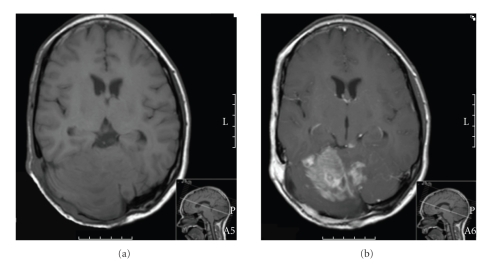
T1-weighted axial MRI-scan of the brain. T1-weighted axial MRI showed a (sub)occipital right-sided bone defect and a lesion in the right cerebellar hemisphere (a) that enhanced after i.v. Gadolinium administration (b).
